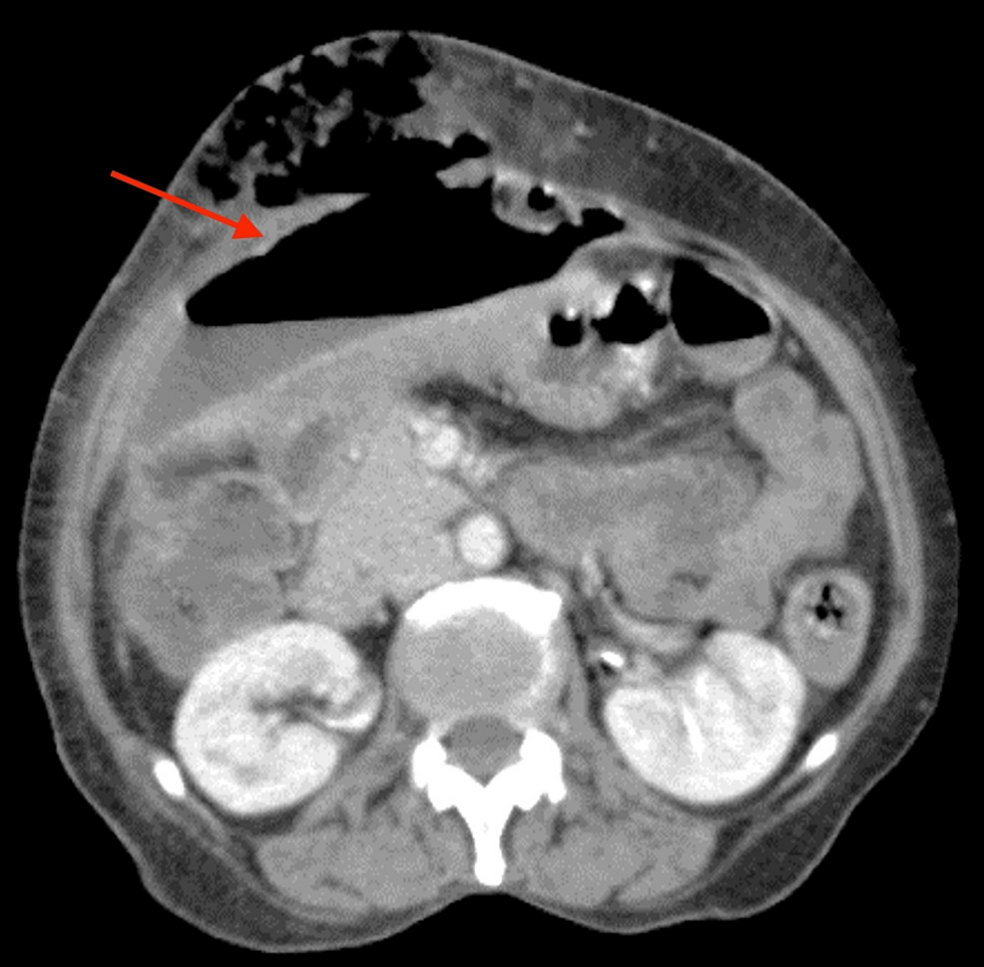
Contrast-enhanced CT showing a collection with enhancing walls The arrow points to air-fluid levels in the costal margin of the right lobe of the liver CT: computed tomography
